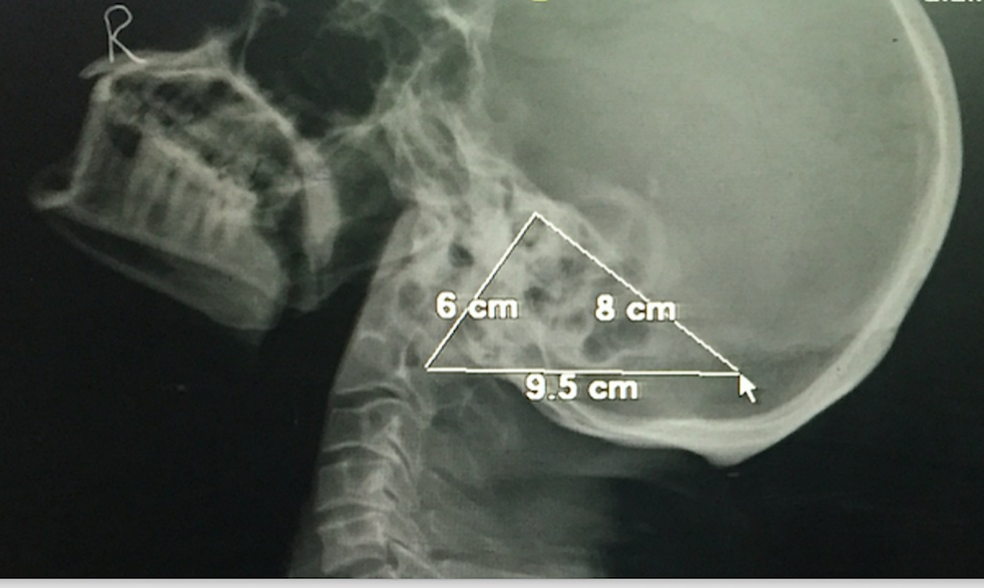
Lateral radiograph of skull showing the mastoid triangle-related measurements
